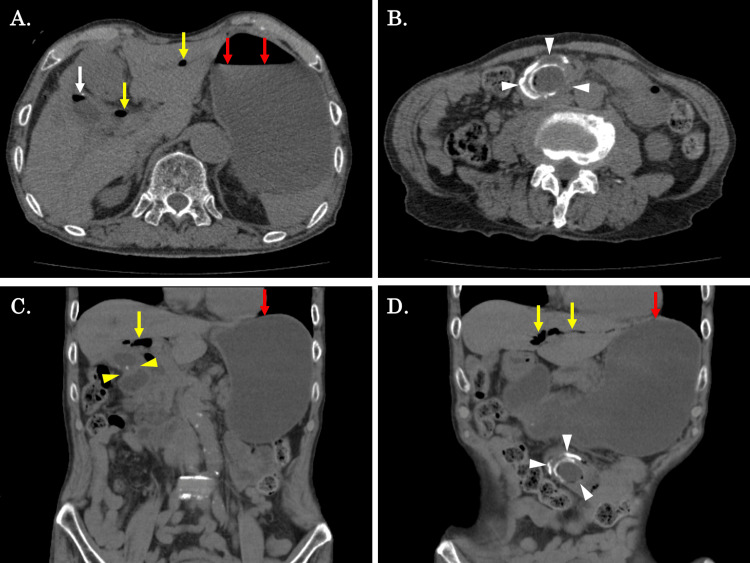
Abdominal CT findings on admission (A, B) An axial CT scan revealed air in the gallbladder (white arrows) and pneumobilia (yellow arrows). The stomach was dilated and filled with fluid (red arrows). A 4 x 3 cm calcified gallstone was located in the third portion of the duodenum (white arrowheads). (C, D) A coronal CT scan showed a cholecystoduodenal fistula (yellow arrowheads). A 4 x 3 cm calcified gallstone was located in the third part of the duodenum (white arrowheads), pneumobilia (yellow arrows), and stomach was dilated and filled with fluid (red arrows), but there was no small bowel dilatation distally.
